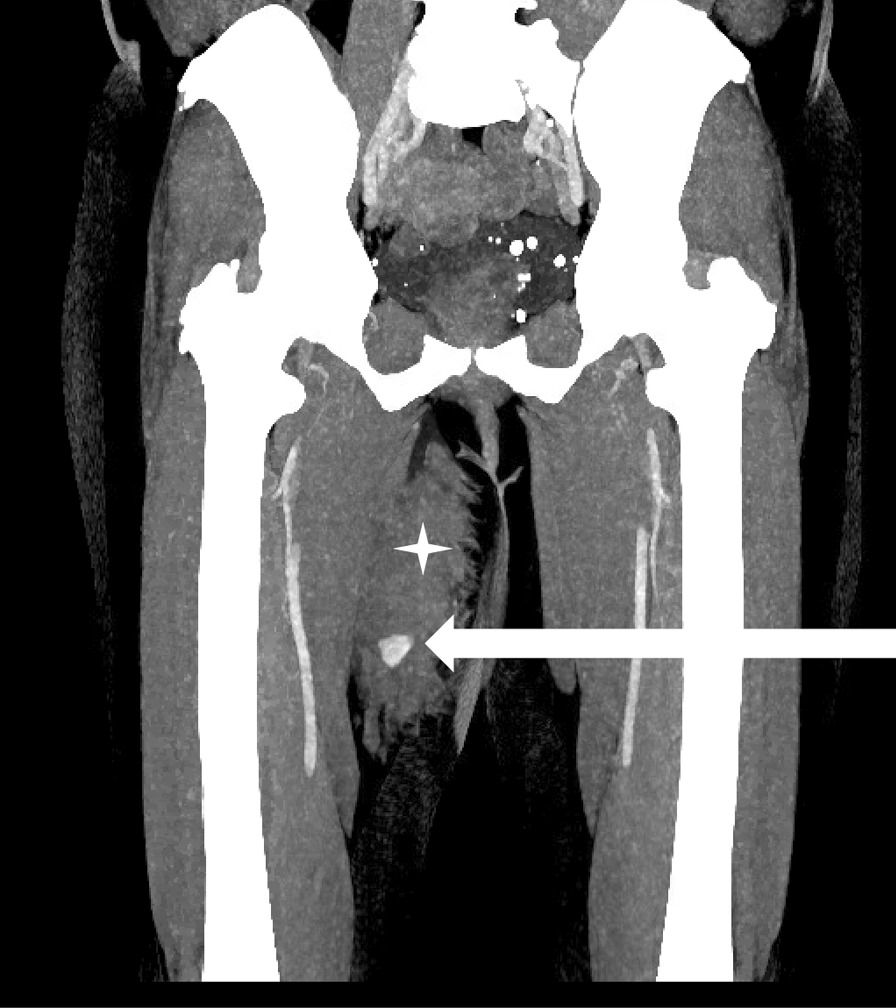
Immediate postoperative contrast-enhanced computed tomography. Coronal view with maximum intensity projection (set at 10 mm) shows a 7-cm hematoma (white star) with a pseudoaneurysm (white arrow). No direct feeding artery is detected
